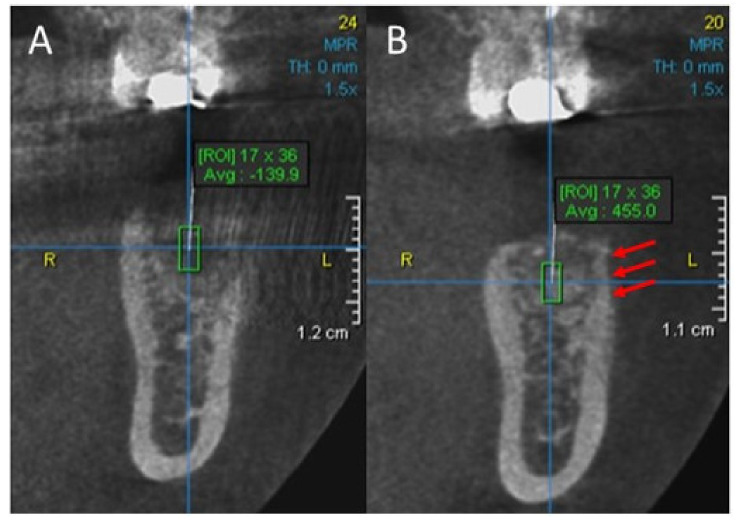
Grey levels in rectangular ROI at the site of augmentation. (A) CBCT scan ten days postoperatively. (B) Cone beam computed tomography (CBCT) scan 6 months post augmentation with ISBG. Pay attention to buccal bone plate regeneration at the site of augmentation (red arrows).
